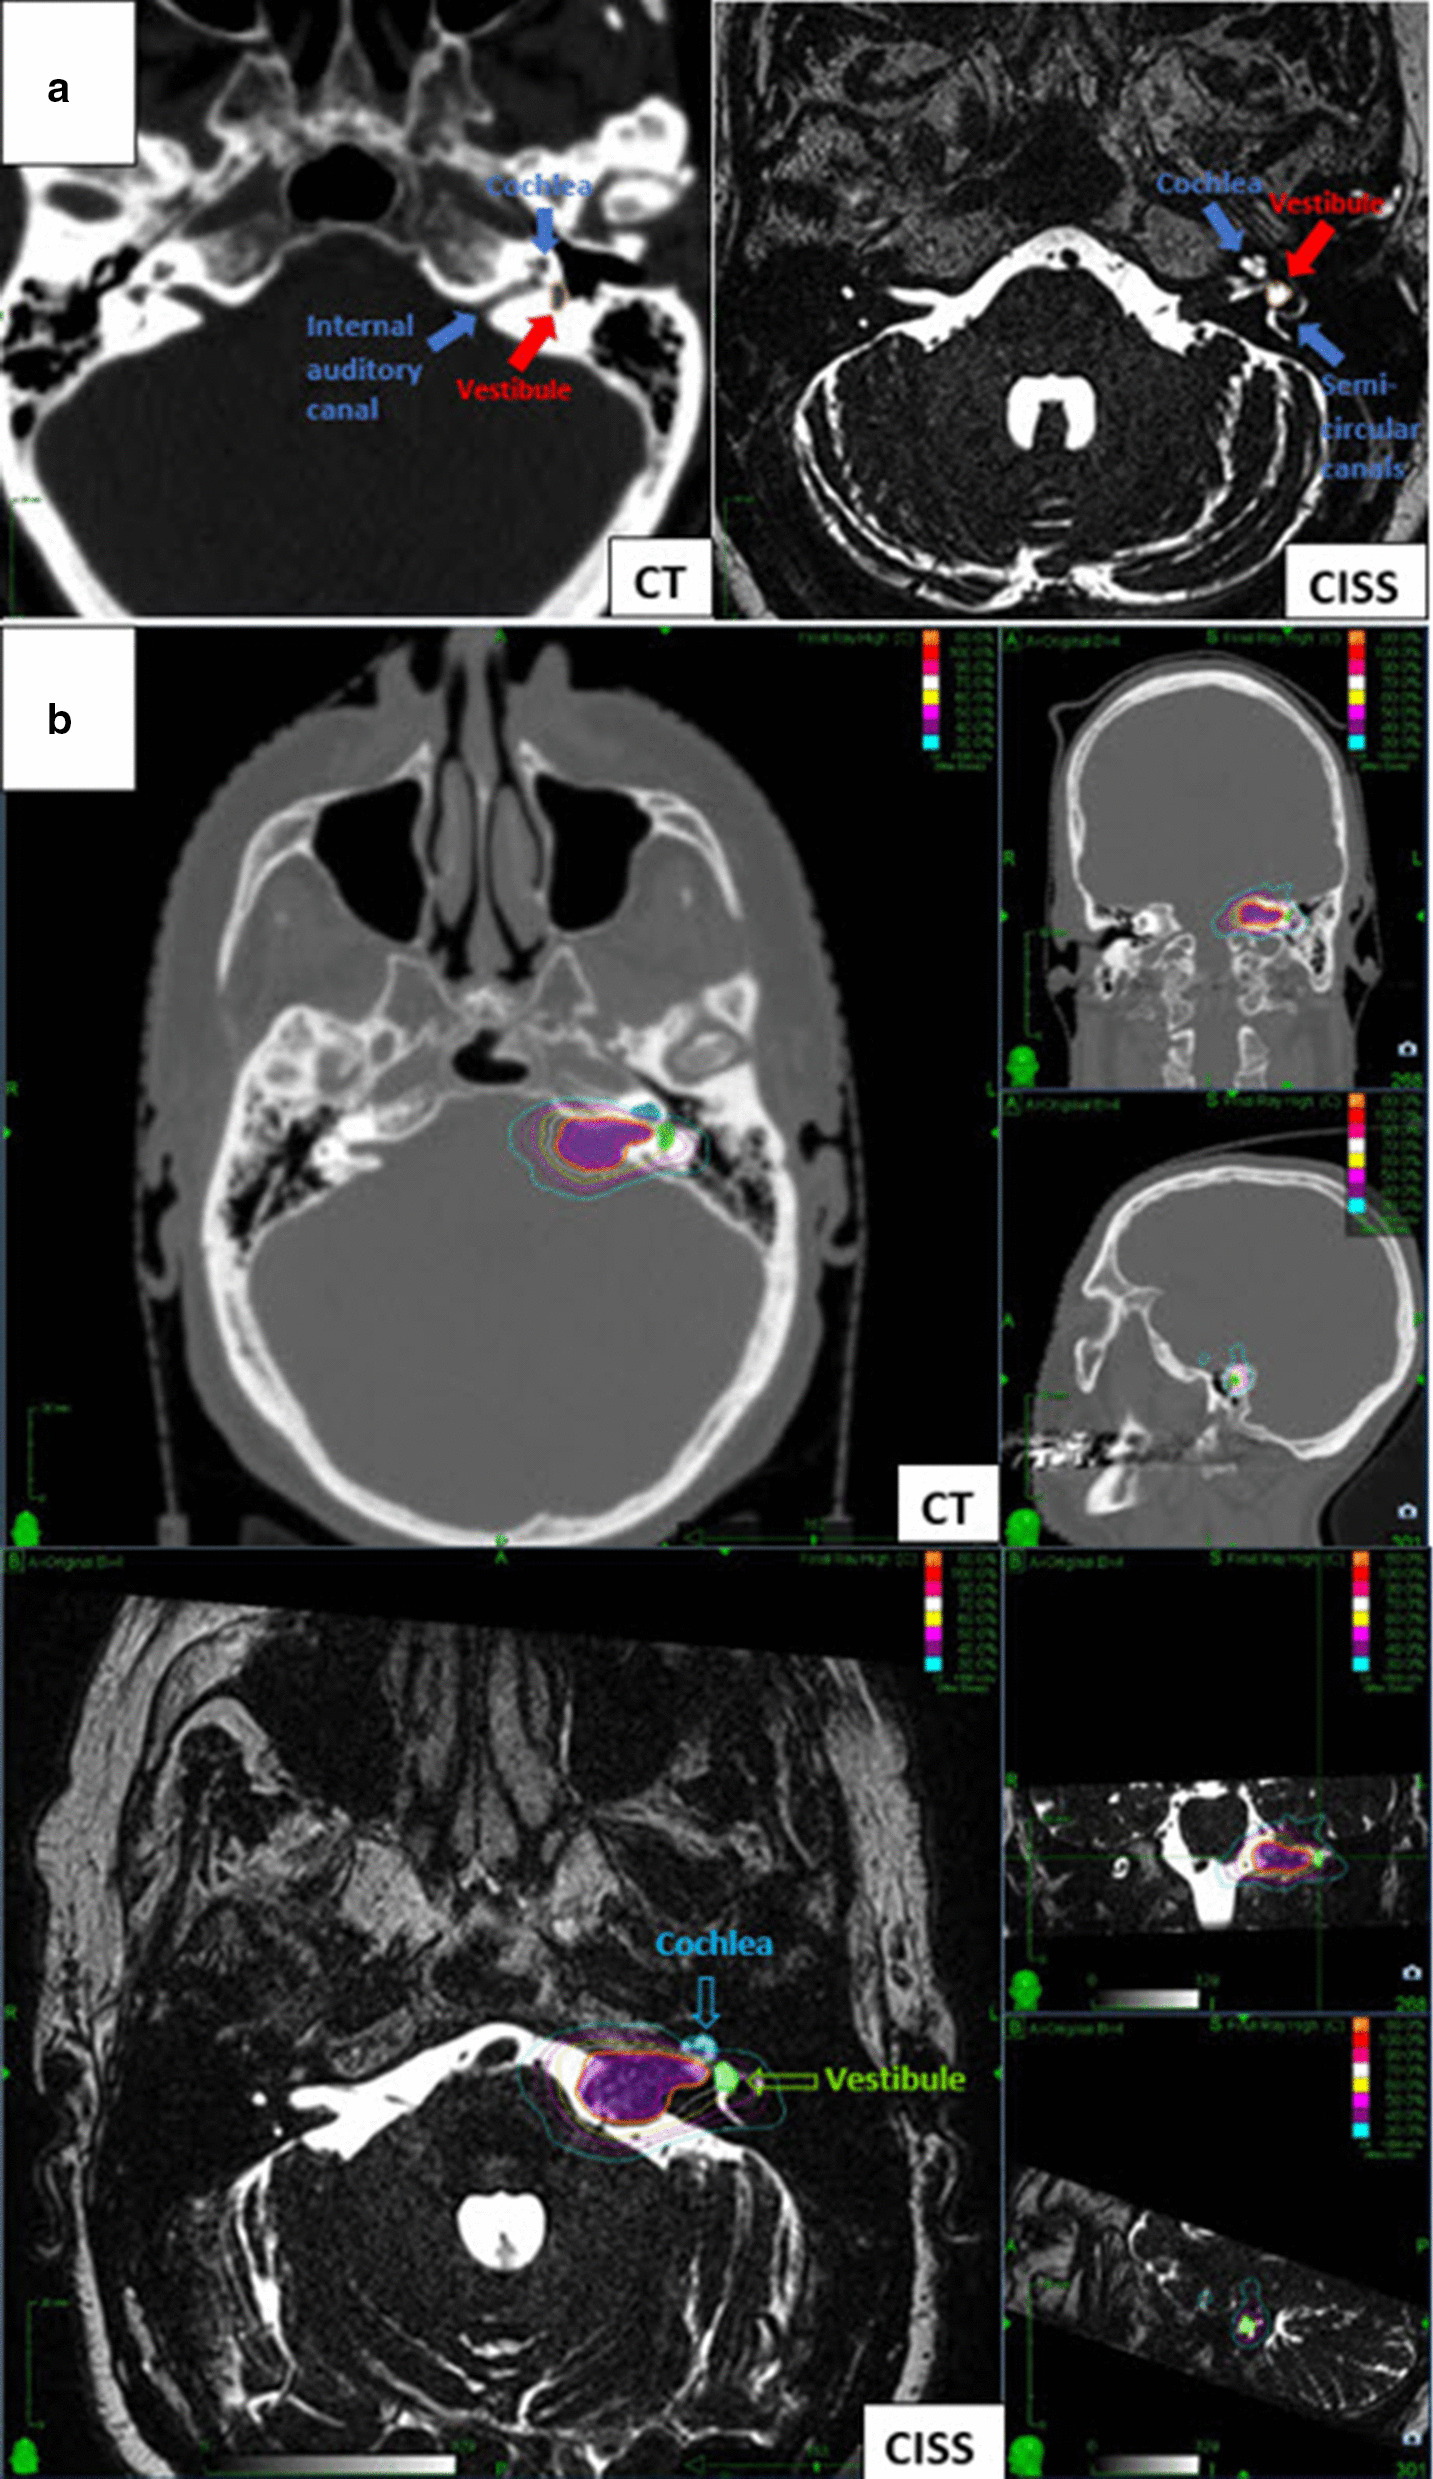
a Axial CT (left) and CISS images showing the volume of vestibule (red arrow, orange line). The blue arrows indicate cochlea, semi-circular canals and internal auditory canal. *CT* computed tomography, *CISS* constructive interference in steady-state. b Axial, sagittal and coronal CISS MR and CT images showing the volume of the cochlea (blue arrow) and vestibule (green arrow) with the different isodose lines from a treatment plan for Cyberknife. A dose of 12 Gy in a single fraction was prescribed to 80% isodose line and no dose constraints were given to the vestibule during treatment plan optimization. It is evident that cochlea is very well spared by giving it priority during plan optimization, while 70% (10.5 Gy) to 30% (4.5 Gy) isodose lines cover the vestibular volume
